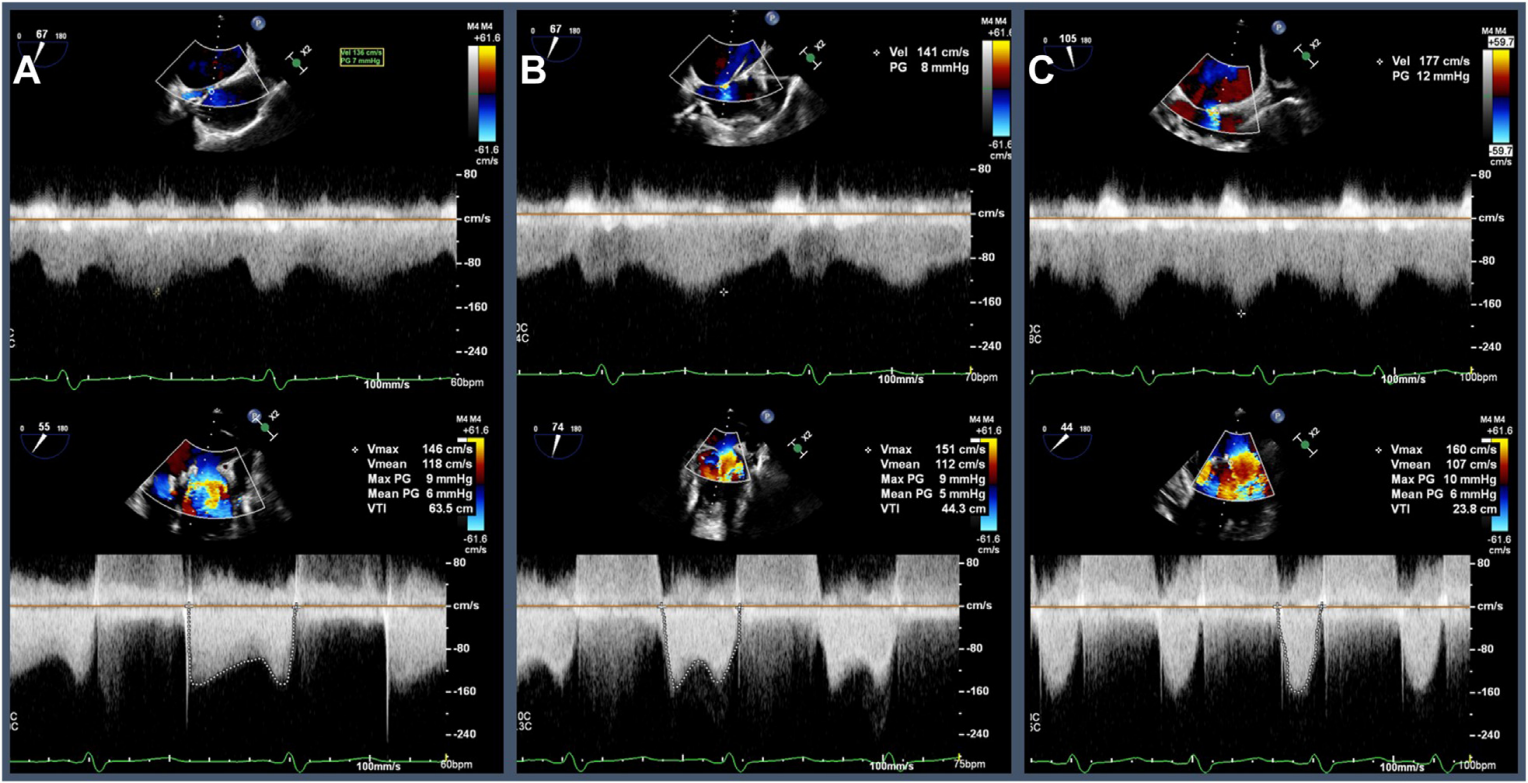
Intraprocedural transesophageal echocardiogram pulse wave Doppler tracings across IAS (top panels) and mitral valve (bottom panels). (A) HR 60 bpm, mean MV gradient 6 mm Hg, IAS velocity 136 cm/s. (B) HR 75 bpm, mean MV gradient 5 mm Hg, IAS velocity 141 cm/s. (C) HR 100 bpm, mean MV gradient 6 mm Hg, IAS velocity 177 cm/s.
